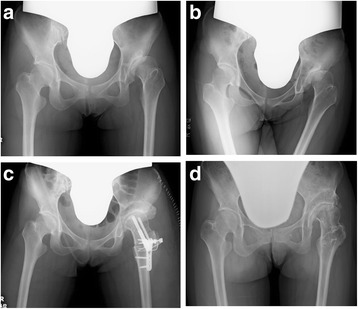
Radiographic image of a 25-year-old woman treated with rotational acetabular osteotomy and valgus intertrochanteric osteotomy on her left hip. The preoperative radiographic image shows AHD with a deformity of the femoral head (a). The joint space becomes wider when the hip is adducted, and the position of the loading area of the femoral head becomes horizontal (b); thus, we performed RAO with valgus femoral osteotomy (c). There is no progression of OA 10 years after the surgery compared with the finding immediately after surgery, and she had no pain or any complaints on her left hip (d)
